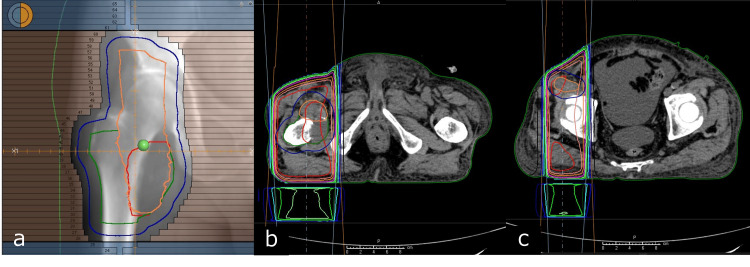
Radiation therapy field and dose distribution RT plan was generated in the Raystation treatment planning system (RaySearch Laboratories AB, Stockholm, Sweden). The radiation therapy fields are displayed in the beam's eye view (a), as well as in the axial images (b,c). The gross tumor volume was defined as the bone metastasis in the popliteal region. The clinical target volume (CTV) was delineated to encompass the area surrounding the femur, including the tumor and the thickened iliopsoas muscle. The planning target volume (PTV) was subsequently defined as the CTV plus an additional 1.0 cm margin. Ten-megavolt (10 MV) X-rays were administered from two anterior and posterior quadrants.
